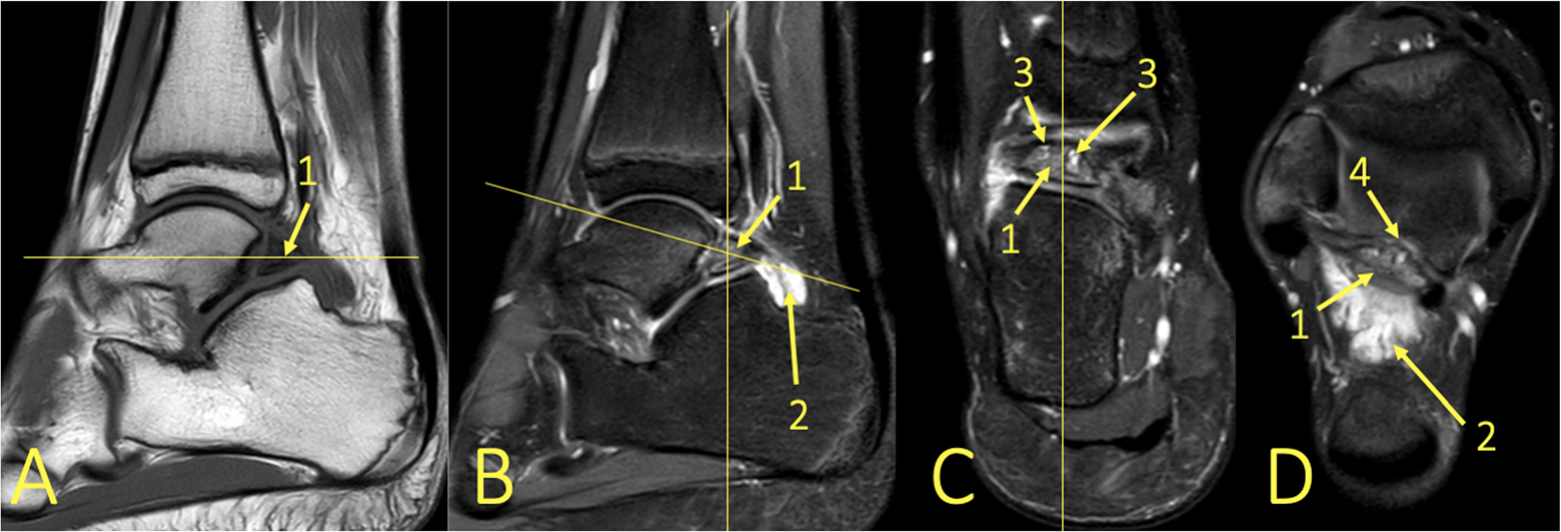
A 16-year-old male with chronic posterior ankle pain and a suspected os trigonum with impingement. (1) MRI showed an os trigonum with BME, (2) effusion and synovitis, and (3) degenerative cysts (4) along the articulation with the posterior talar process
